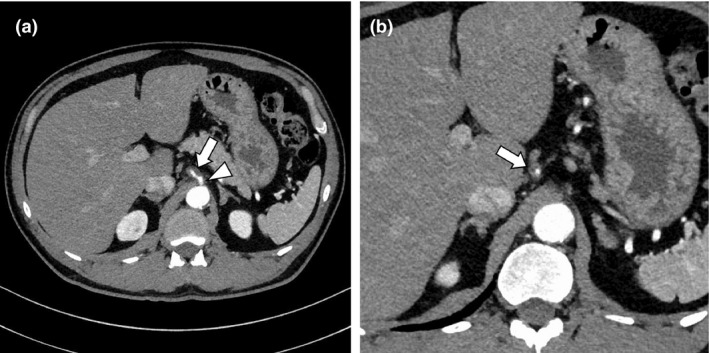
Abdominal contrast‐enhanced CT on admission. Low‐attenuated area was shown around the left gastric artery (a, b, arrows) arising from the abdominal aorta (a, arrowhead).
